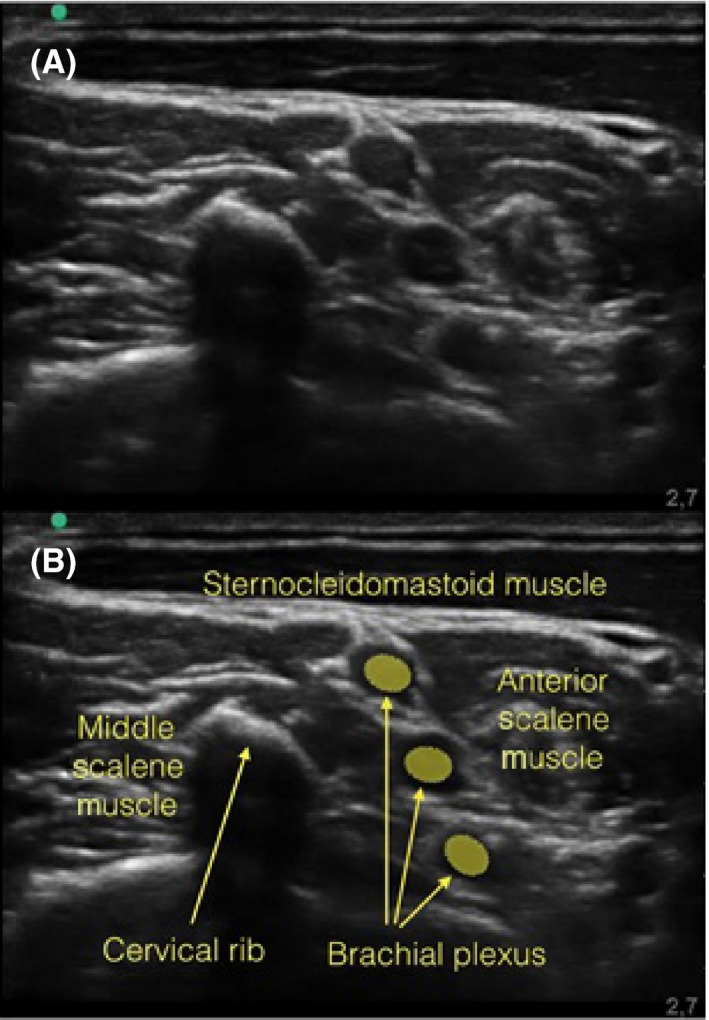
Interscalene approach of brachial plexus. A, The ultrasound of the interscalene space. B, The same picture with labels of the anatomical structures
